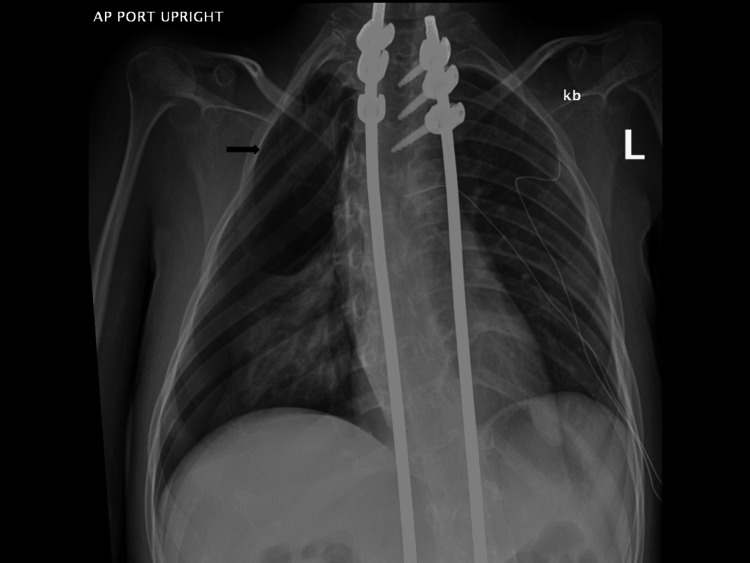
Chest radiograph of the second right-sided pneumothorax more extensive involving the right upper lobe (black arrow).
